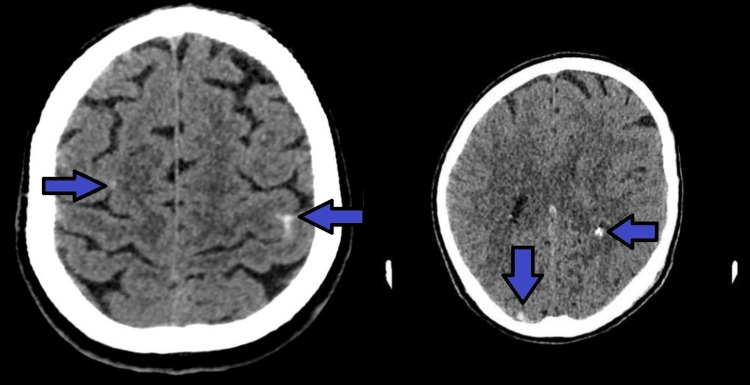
Non-contrast cranial CT scan showing subarachnoid hemorrhage (blue arrows). Image quality limited by patient non-cooperation.
